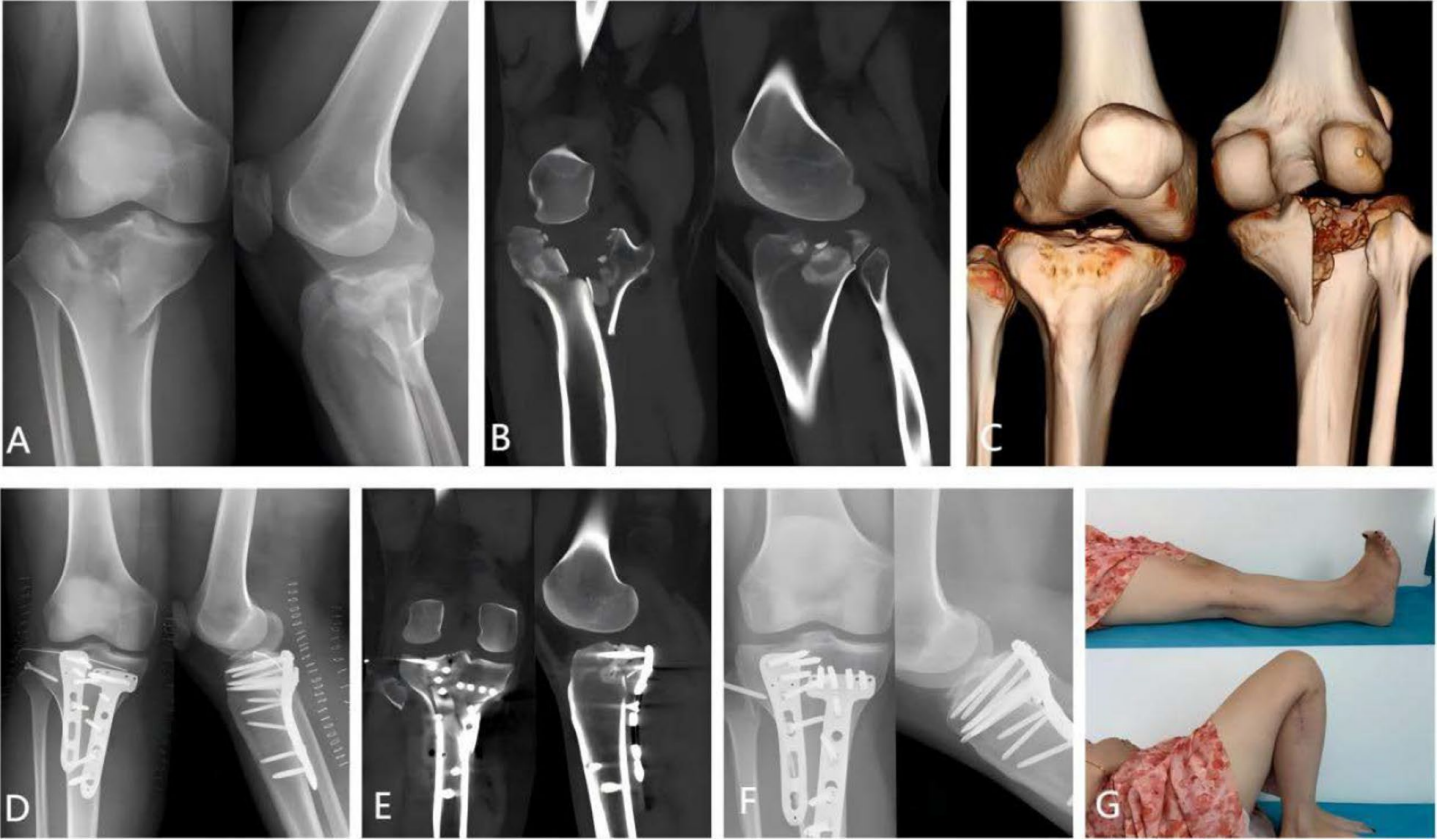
A patient with bilateral tibial plateau fractures, classified as Type V PL + PM + AM according to the Kfuri–Schatzker classification. A Preoperative anteroposterior and lateral radiographs of the affected knee. B, C Preoperative CT scans and 3D reconstruction of the affected knee, showing significant collapse of the posterolateral joint surface, rupture of the posterior wall, and fracture of the medial column. D Postoperative anteroposterior and lateral radiographs of the affected knee, demonstrating perfect fracture reduction. E Postoperative CT scans and 3D reconstruction of the affected knee, showing excellent fracture reduction. F Anteroposterior and lateral radiographs at 6-month follow-up, indicating complete healing of the fracture and osteotomy site. G Radiographs of the affected knee at 1-year follow-up, showing excellent recovery of knee function. *AM* anteromedial, *PM* posteromedial
